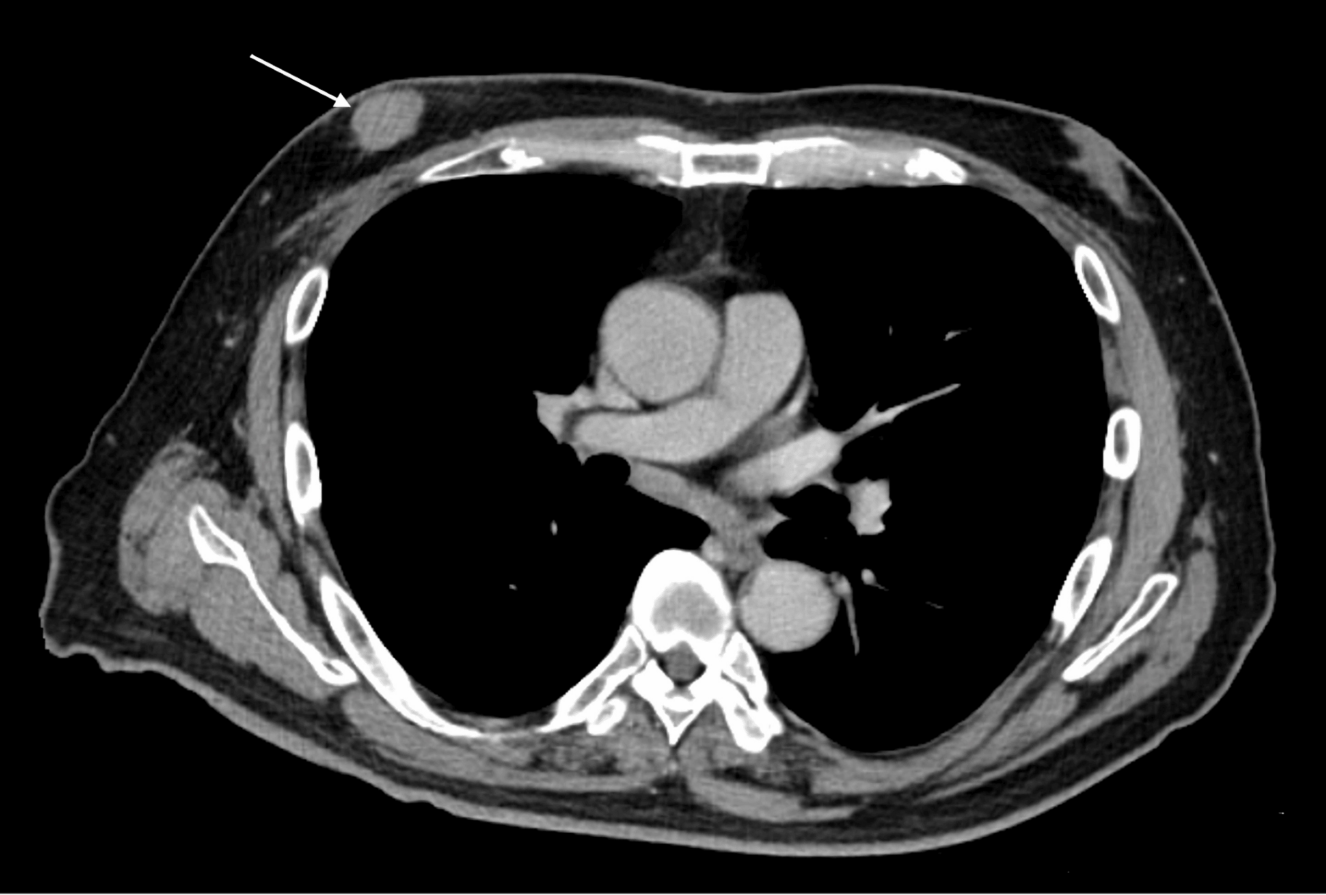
Contrast-enhanced axial chest computed tomography on initial presentation Contrast-enhanced axial chest computed tomography image showing a well-defined, homogeneously enhancing 2.3-cm mass located immediately beneath the right nipple, representing the primary breast tumor.
